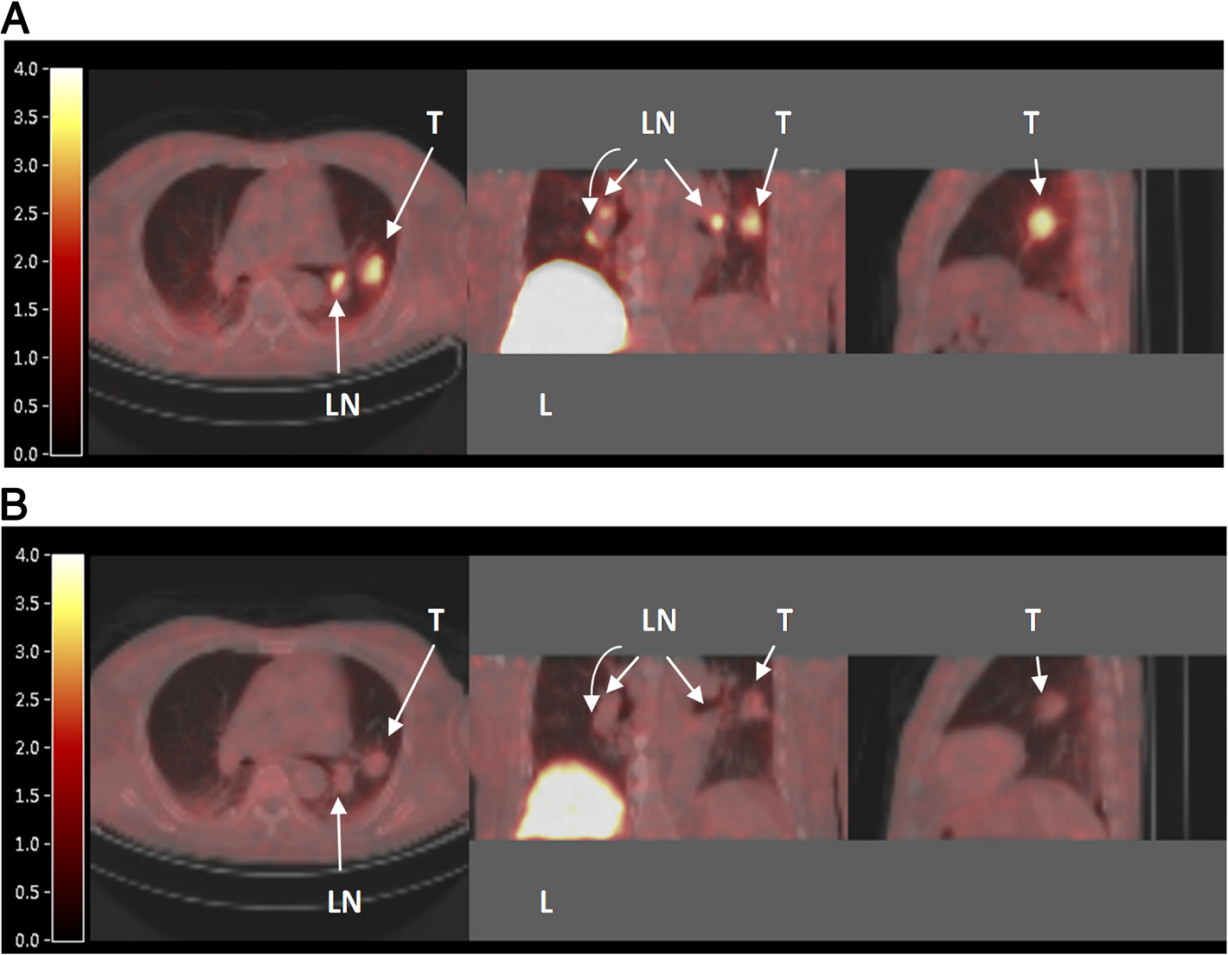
[^11^C]Erlotinib PET images. PET images of a typical patient (Nr 8), who was scanned first off erlotinib therapy (a); he then started therapy and was scanned again after 7 days (b). Axial, coronal, and sagittal views are shown, obtained by CT-fused parametrically reconstructed [^11^C]erlotinib TBR_art,50–60_ PET images. The color scale indicates the TBR_art,50–60_ value per pixel (unitless). The primary tumor lesion (*T*) and regional lymph nodes (*LN*) are clearly visible in the absence of erlotinib therapy. Also, high uptake is seen in the liver (*L*)
